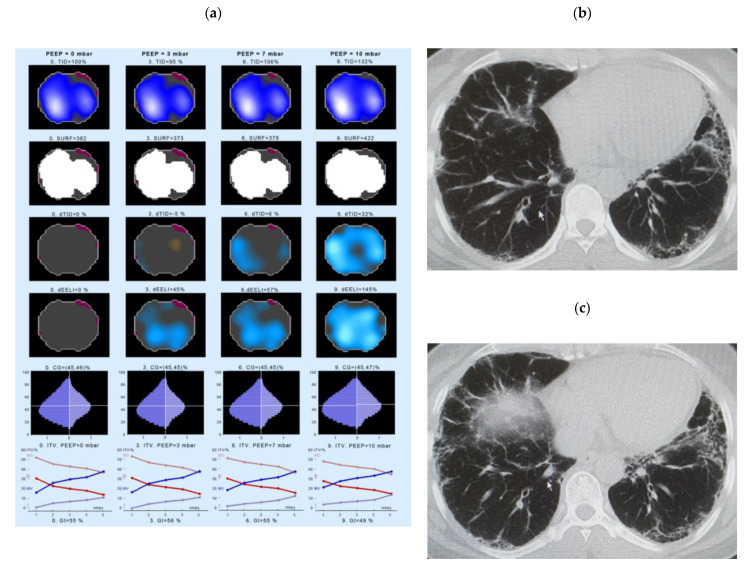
Changes in regional lung ventilation in IPF patient #4. Left panel (a): Spatial distribution of TID, SURF, dTID, dEELI in the electrical impedance (EI) tomogram at a PEEP of 0 (left), 3 (2nd left), 7 (3rd from left), and 10 cm H_2_O levels (right column). The lowest panel indicates the intratidal gas distribution (ITV) for four anatomical areas during one respiration. Given are the distribution of ventilation into the ventral (V), dorsal (D), medio-ventral (MV) and medio-dorsal (MD) regions of the lung. The X scale (1–5) shows the entire inspiration, divided into 5 steps. Right panel (b,c): The respective high resolution CT picture corresponding to the ROI of EIT measurement is shown. Abbreviations: CG—center of gravity of ventilation distribution; dEELI—difference of end-expiratory lung impedance over time; ITV—intratidal gas distribution; PEEP—positive end-expiratory pressure; SURF—surface of ventilated areas, TID—tidal impedance distribution; dTID—difference of TID over time.
